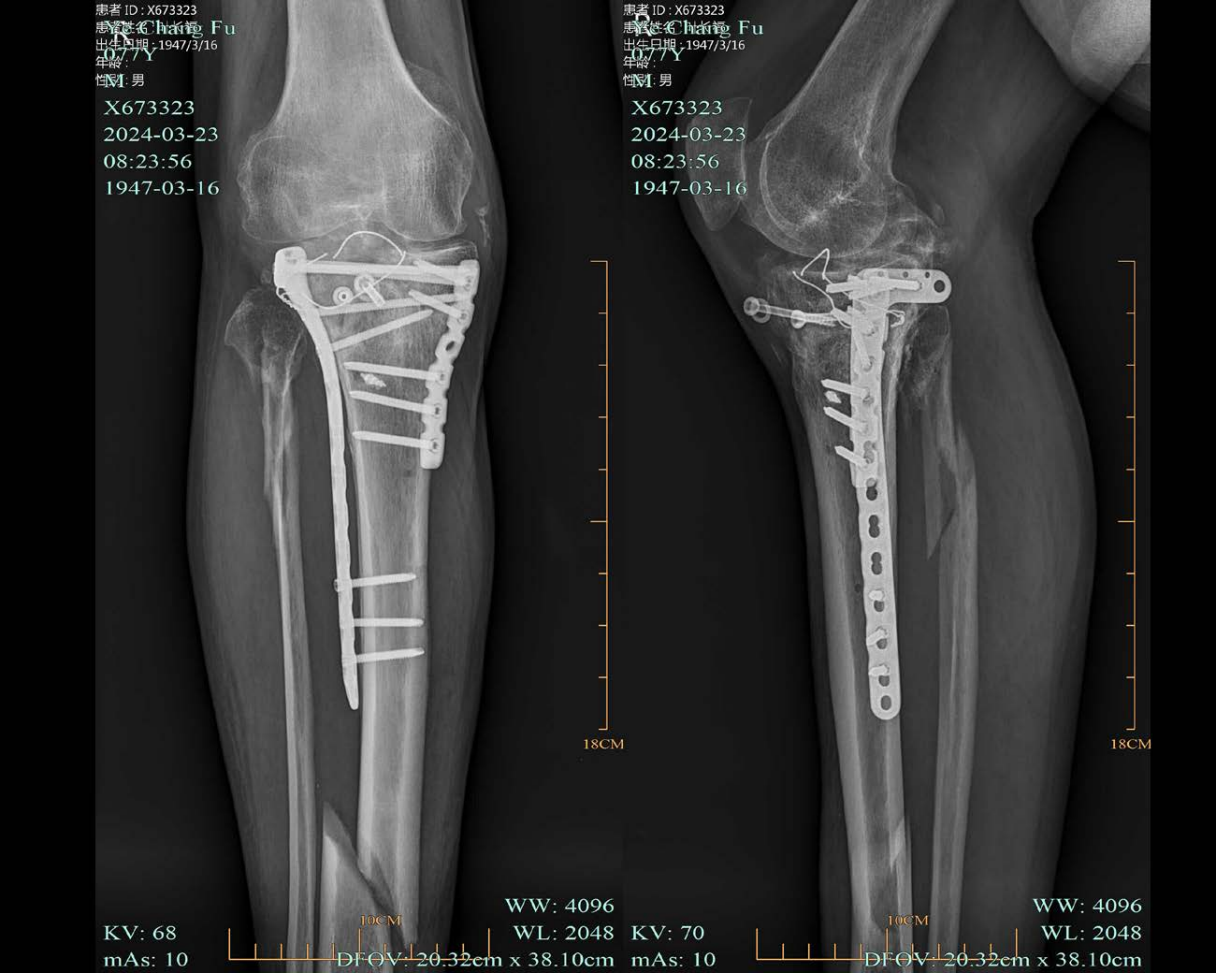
Radiography scan showed wire snap and loosened screw (Fig. 9; date of examination: March 23, 2024). (A) Plain x-ray of the knee with anteroposterior view. (B) Plain x-ray of the knee with lateral view.
